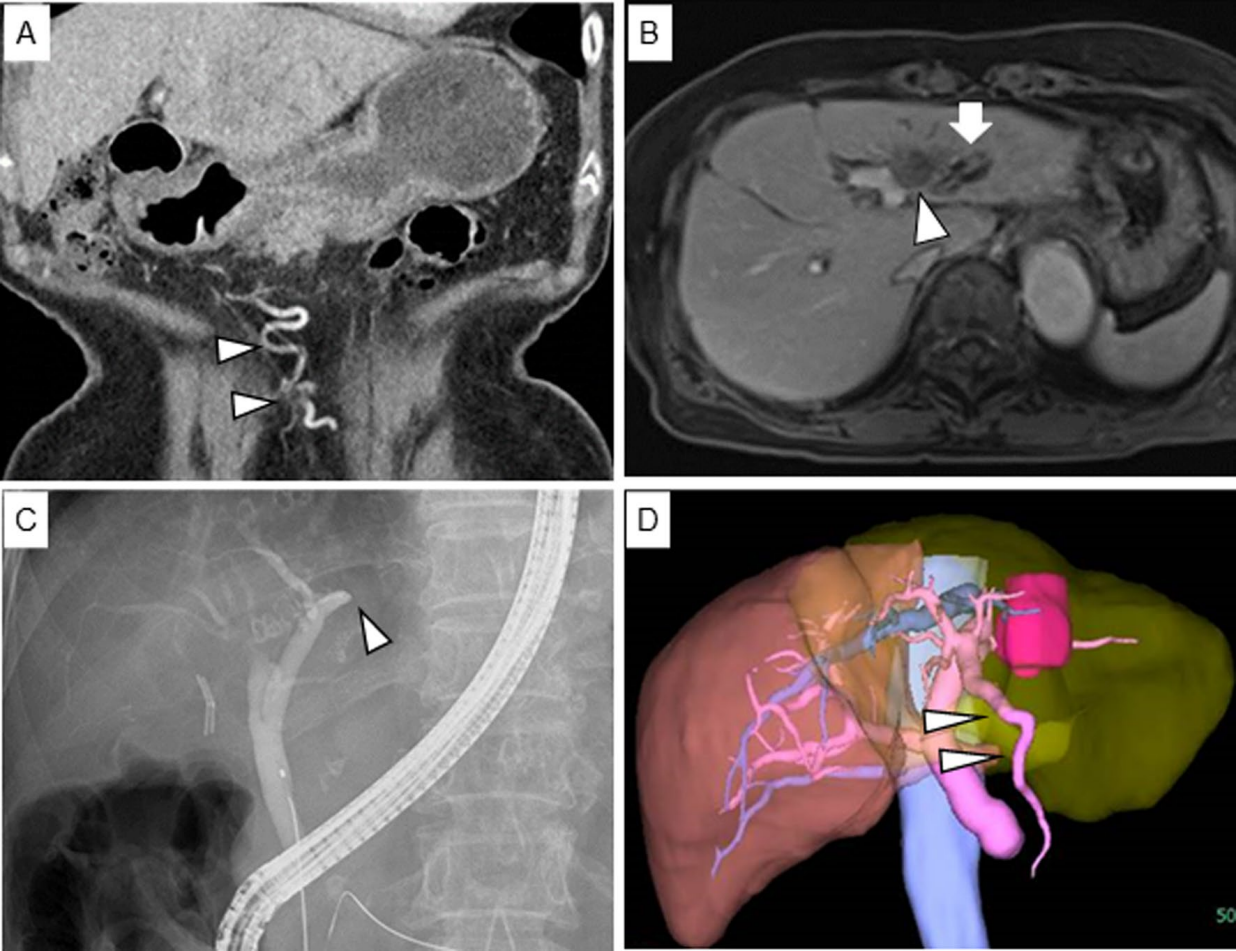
Computed tomography revealed development of paraumbilical vein (A, arrowhead). Magnetic resonance imaging revealed low-density tumor in contact with umbilical portion (B, arrowhead) and shows bile duct dilation (B, arrow). Endoscopic retrograde cholangiography showed that the left intrahepatic bile duct was disrupted (arrowhead) and there was obstructive jaundice due to tumor compression (C). Three-dimensional volumetry shows the remaining liver volume is 53% (D) and revealed development of paraumbilical vein (D, arrowhead)
